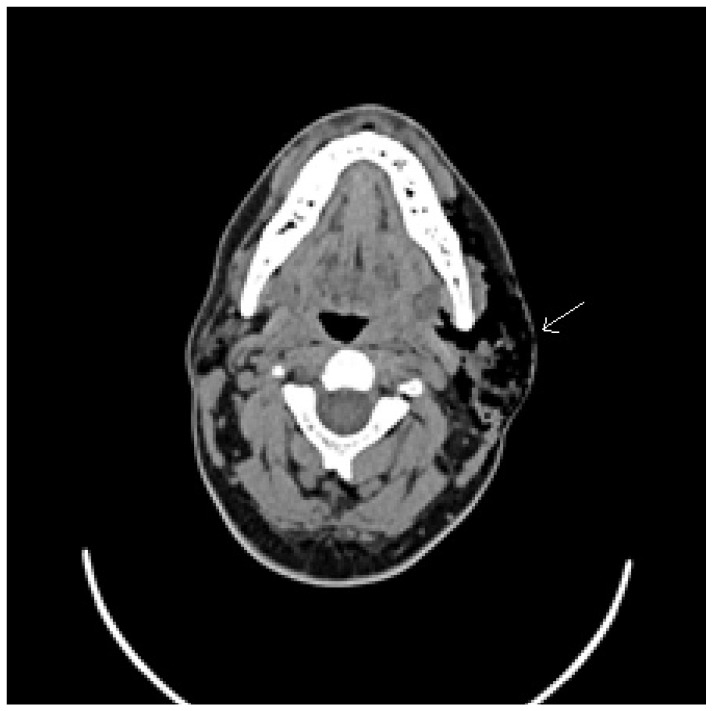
Axial projection computed tomography (CT) image of a left pneumoparotid case, with the arrow indicating the presence of air in the parotid lodge.
